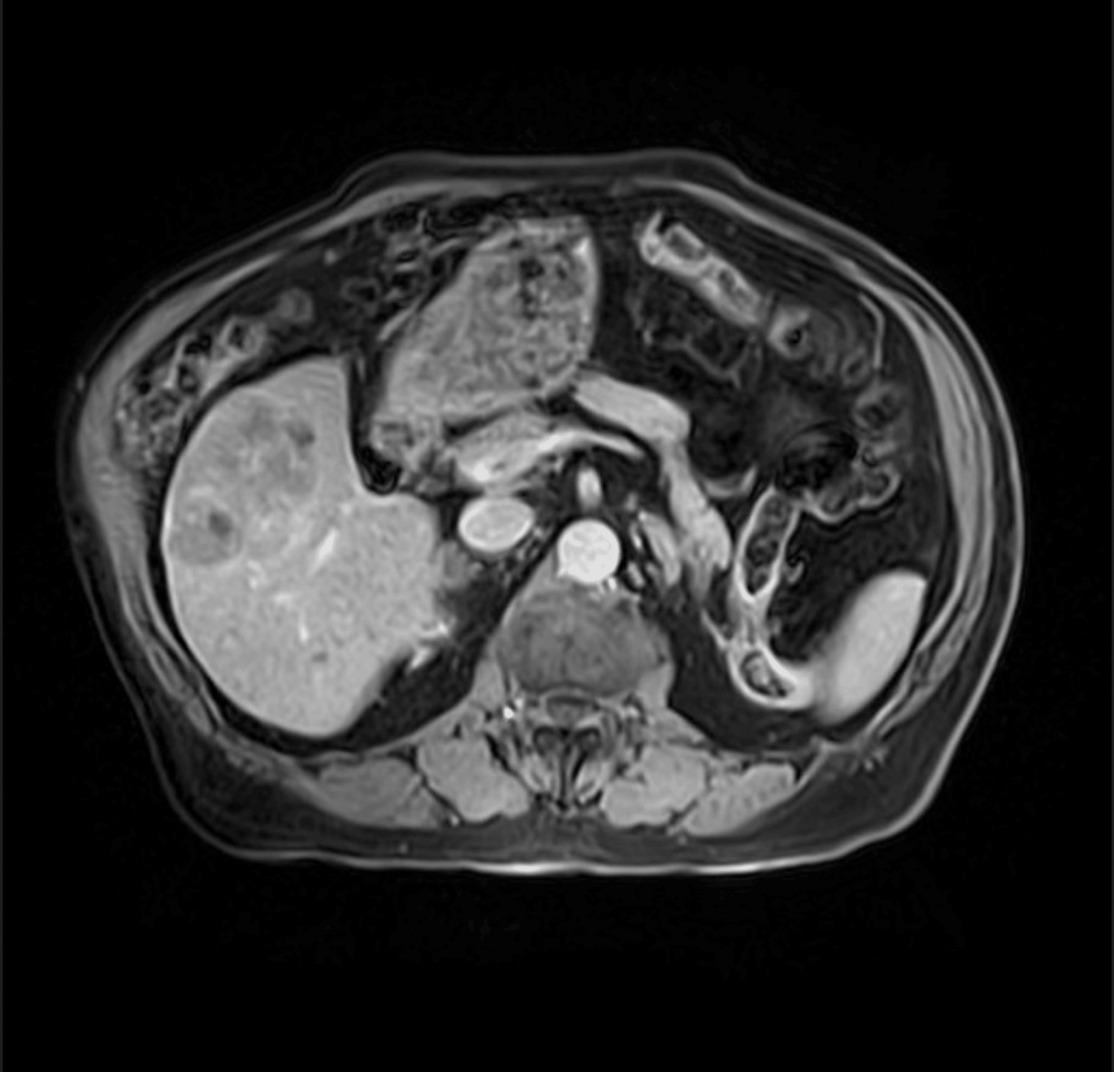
An MRI of the abdomen showing primary hepatocellular carcinoma with no intra-abdominal metastasis
